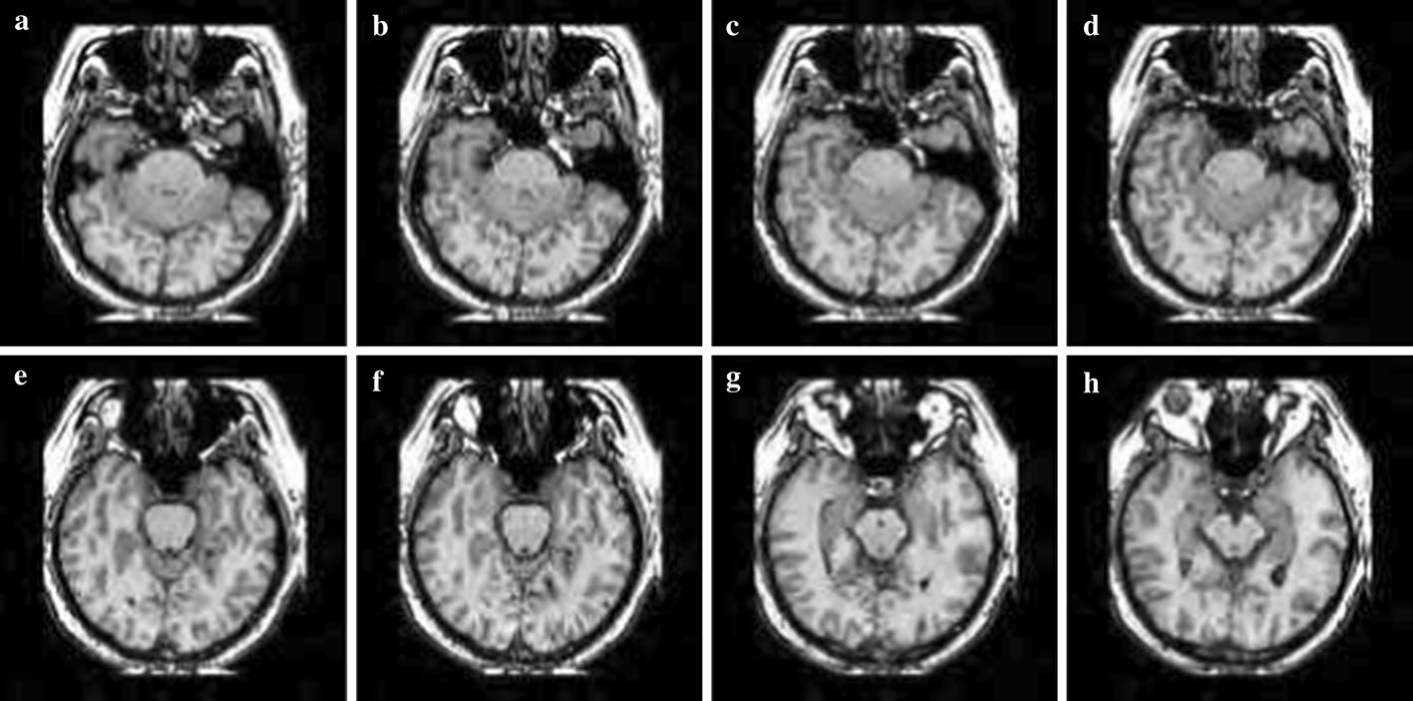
Compressed MRI image slices (a-h) by 3D-SPIHT at a BR = 1.0 bpp
